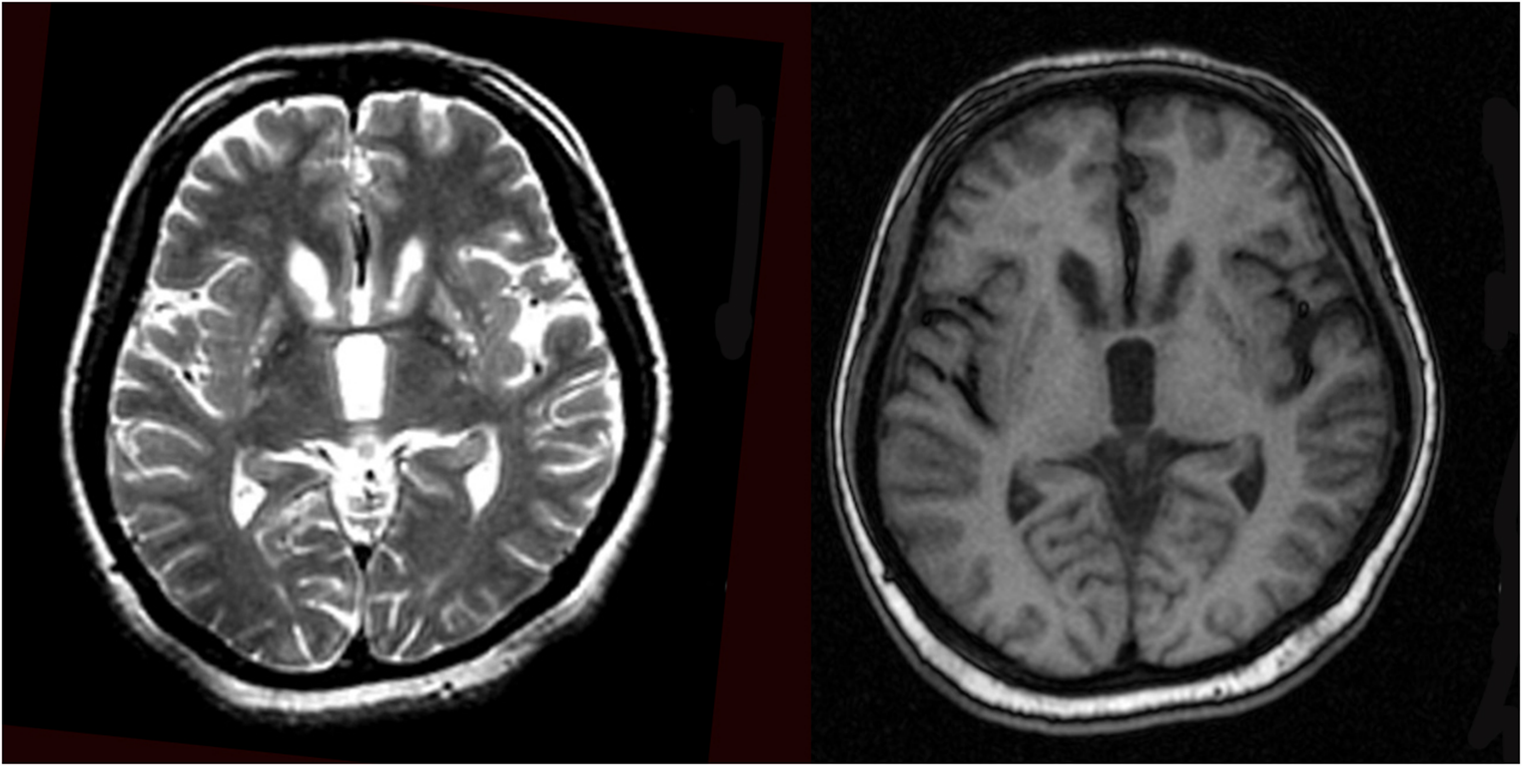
MRI of patient demonstrating bilateral striatal atrophy and degeneration
